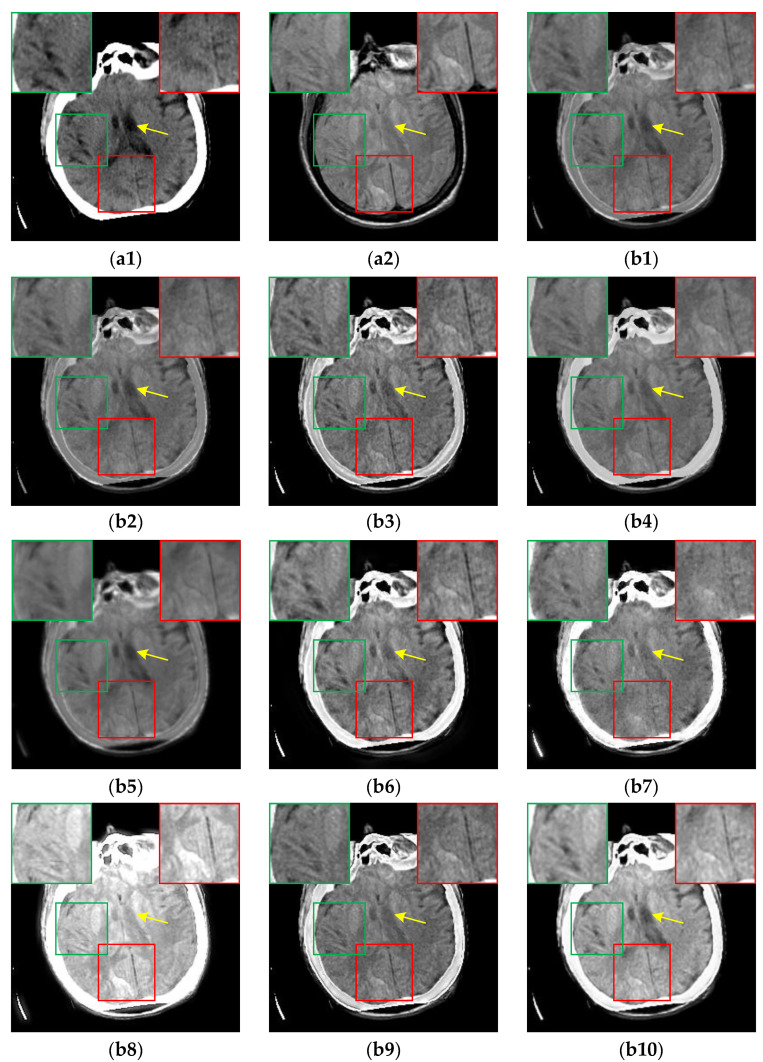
The results of all algorithms on the CT-MR image pairs from Atlas dataset. (a1) Source CT image; (a2) Source MR image; (b1) U2Fusion; (b2) DenseFuse; (b3) IFCNN; (b4) NestFuse; (b5) RFN-Nest; (b6) PAPCNN; (b7) ReLP; (b8) TIF; (b9) CDDFuse; (b10) CIRF.
